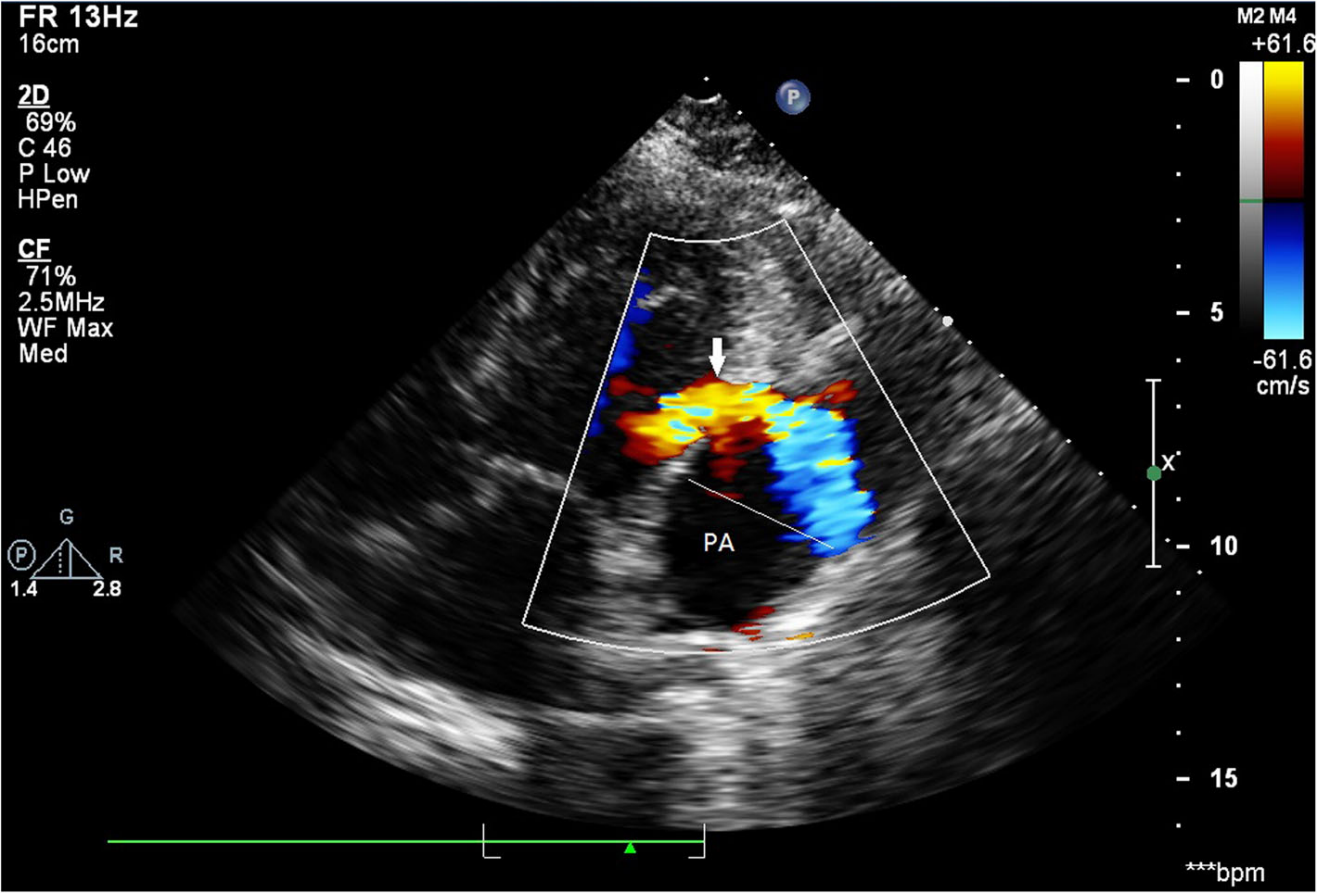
Doppler echocardiography demonstrated to-and-fro singals in a myocardial defect (11 mm) in the lateral wall (indicated by the arrow) and the formation of pseudoaneurysm (PA: pseudoaneurysm)
